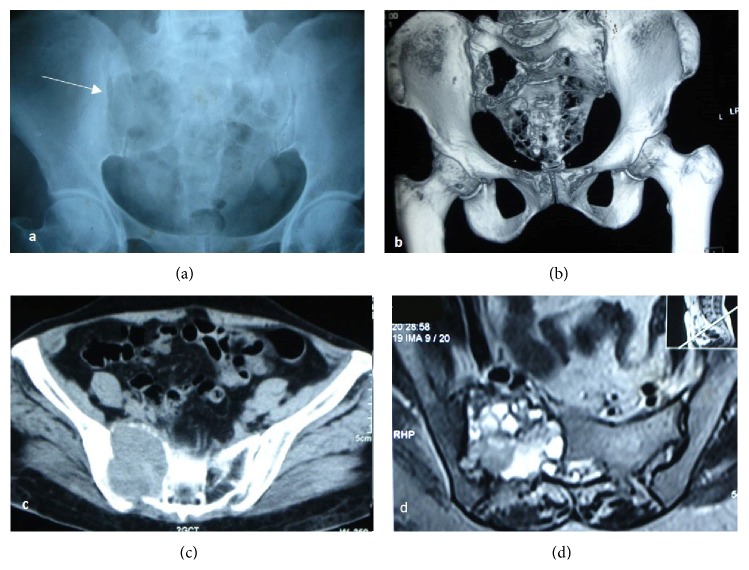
(a) Radiograph of the pelvis AP view; (b) 3D reconstruction and (c) axial section of CT showing expansile lytic lesion involving the right sacral ala with cortical break and surrounding soft-tissue component extending into right SI joint space and pelvis measuring 5.2 × 5.7 × 6.3 cm eroding the adjacent iliac bone. Mass extending into the sacral canal. (d) MRI showing large expansile heterogeneous lobulated lesion with few septations involving right sacroiliac joint. Predominantly lytic lesion with cortical destruction features suggestive of GCT.
